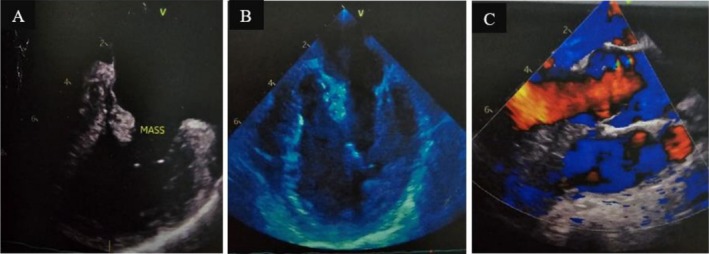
Photograph of trans‐esophageal echocardiogram demonstrated a pedunculated mass attached to the near insertion point of AML into the left ventricle during diastole, suggestive of LA myxoma. There were no vegetation/thrombus (A, B). Eccentric moderate mitral regurgitation was noted (C). LA, lupus anticoagulant.
